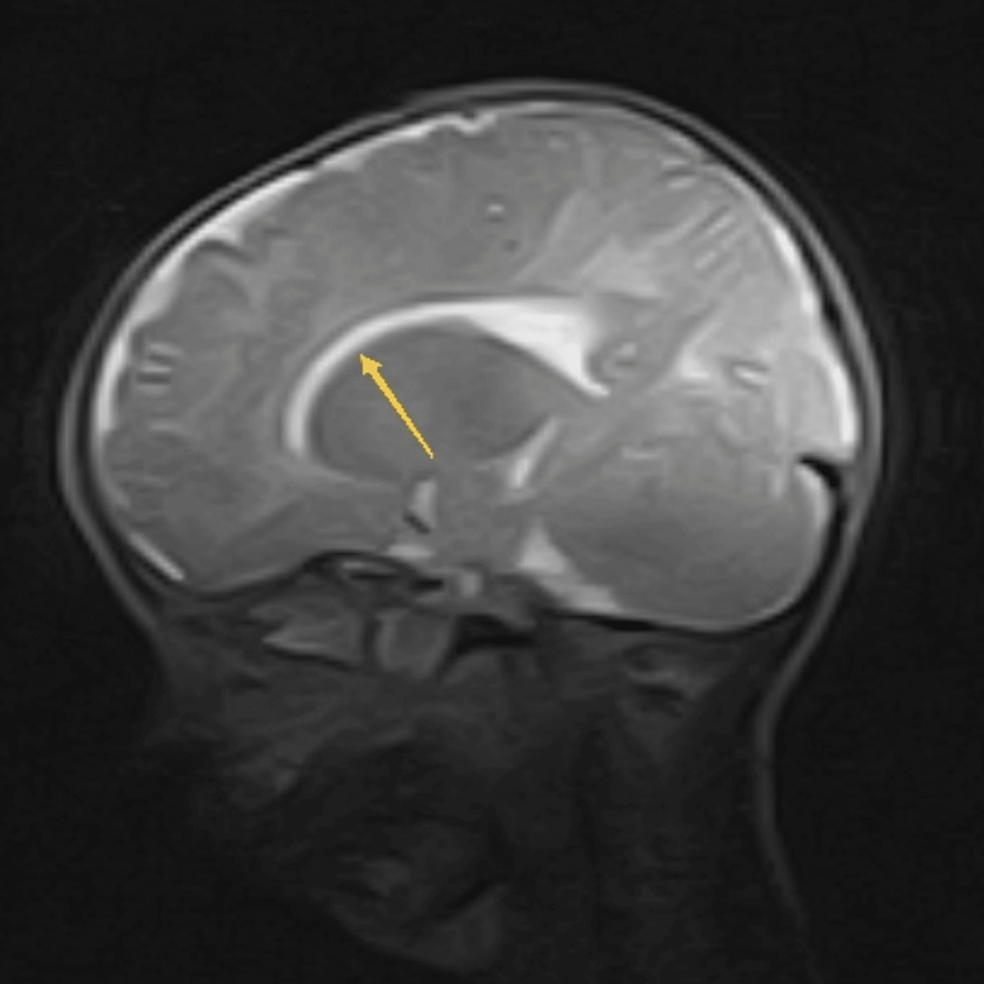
MRI brain T2W image shows thinning of corpus callosum (yellow arrow). MRI: Magnetic resonance imaging; T2W image: T2-weighted image
